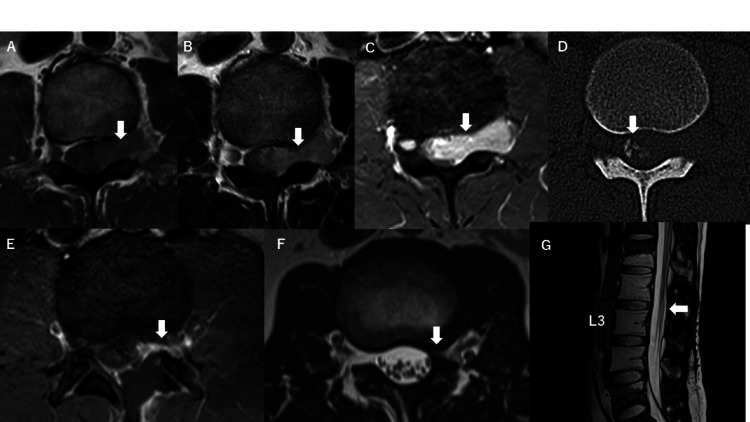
Preoperative and postoperative images. There is a dumbbell-shaped tumor with transforaminal extension at the left L2-3 level. It appears (A) homogeneously isointense (arrow) on the T1-weighted image, (B) slightly heterogeneously hyperintense (arrow) on the T2-weighted image, and (C) shows homogeneous enhancement (arrow) on gadolinium-diethylenetriamine pentaacetic acid (Gd-DTPA). (D) CT shows a calcified portion (arrow) in the tumor (D). (E) MRI (axial) showing the presence of residual tumor (arrow) after surgery on Gd-DTPA. (F, G) MRI showing no evidence of recurrence (arrow), five years after surgery on the T2-weighted image.
